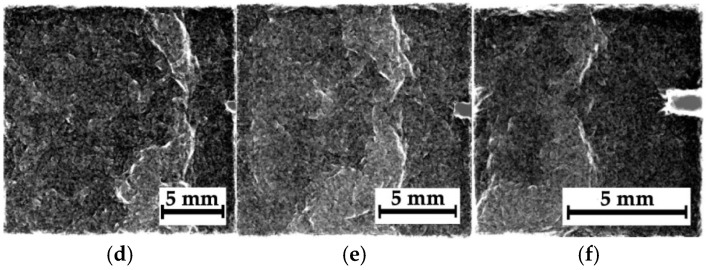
The results of processing of the radiographic images shown in Figure 11 as the phase variation ones (a–c), as well as the mixtures of the original images and the phase variations (d–f). The distances between the sample and the sensor are 39 mm (a,d), 160 mm (b,e), and 240 mm (c,f).
